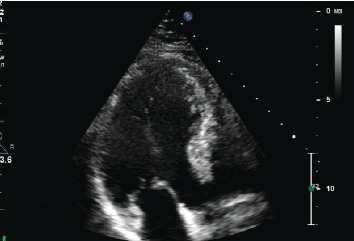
Subsequent echocardiogram revealing a completely recovered myocardium.
